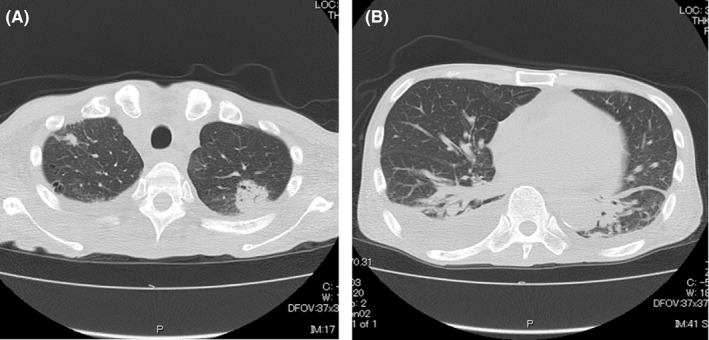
A, Chest CT findings. Organizing pneumonia was observed in both upper lobes. B, Chest CT findings. Bilateral pleural effusion was present
